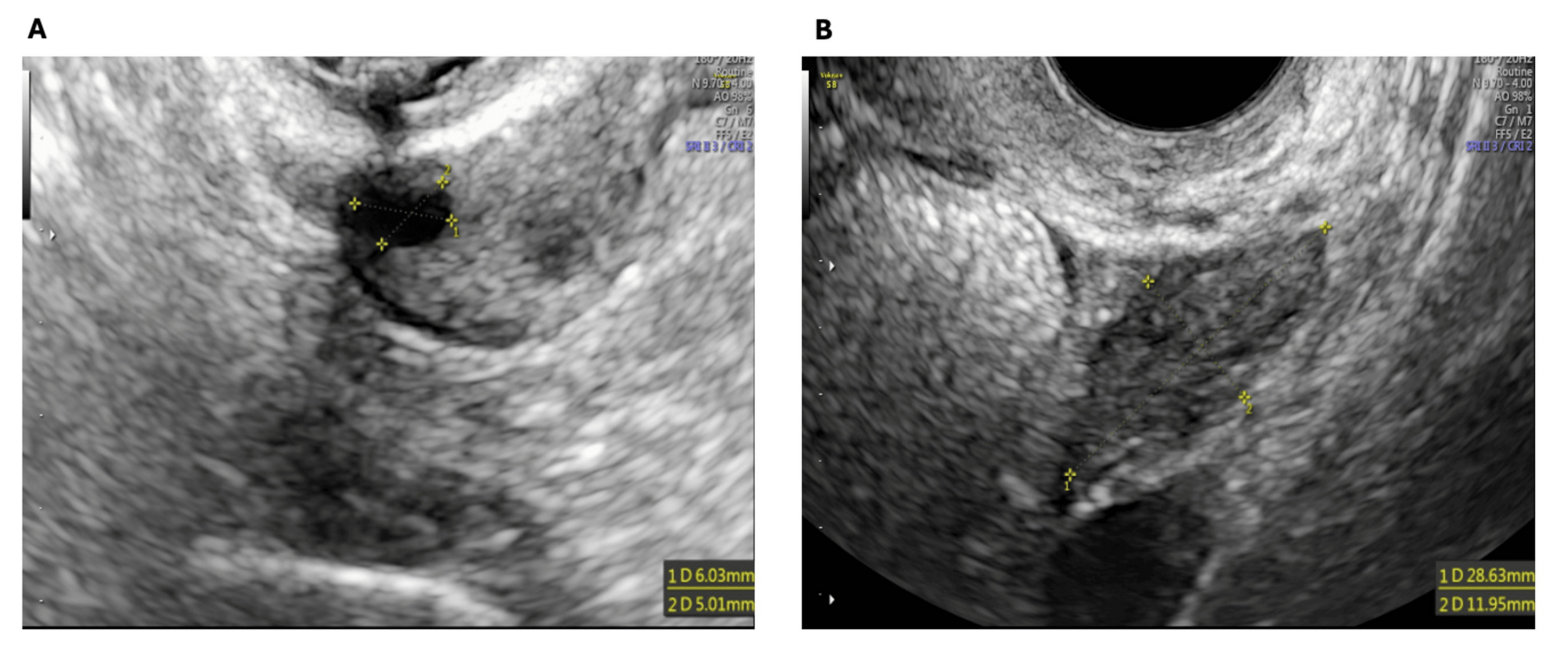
Ovarian ultrasound findings one month after the first PRP treatment. (A) Transvaginal ultrasound of the right ovary showing a single antral follicle measuring 6 mm in diameter at one month post-initial PRP administration. (B) Transvaginal ultrasound of the left ovary demonstrating absence of antral follicles at the same time point. PRP: platelet-rich plasma
